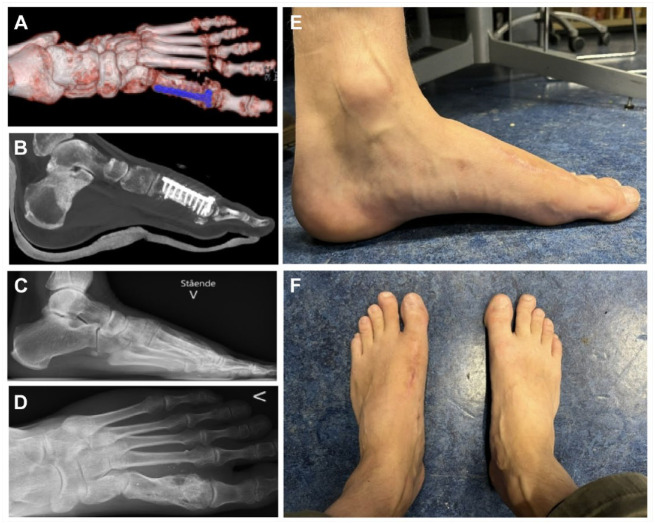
The figure shows the postoperative computed tomography scan, with the bone graft and the metal plate for fixation (4A and B), the lateral and anterioposterior radiographs show the left foot 1 year after iliac graft implantation with integration of the graft (4C and D), the left foot from medial view (4E), and the left and right foot from the anterior view (4F) 23 months after surgery.
